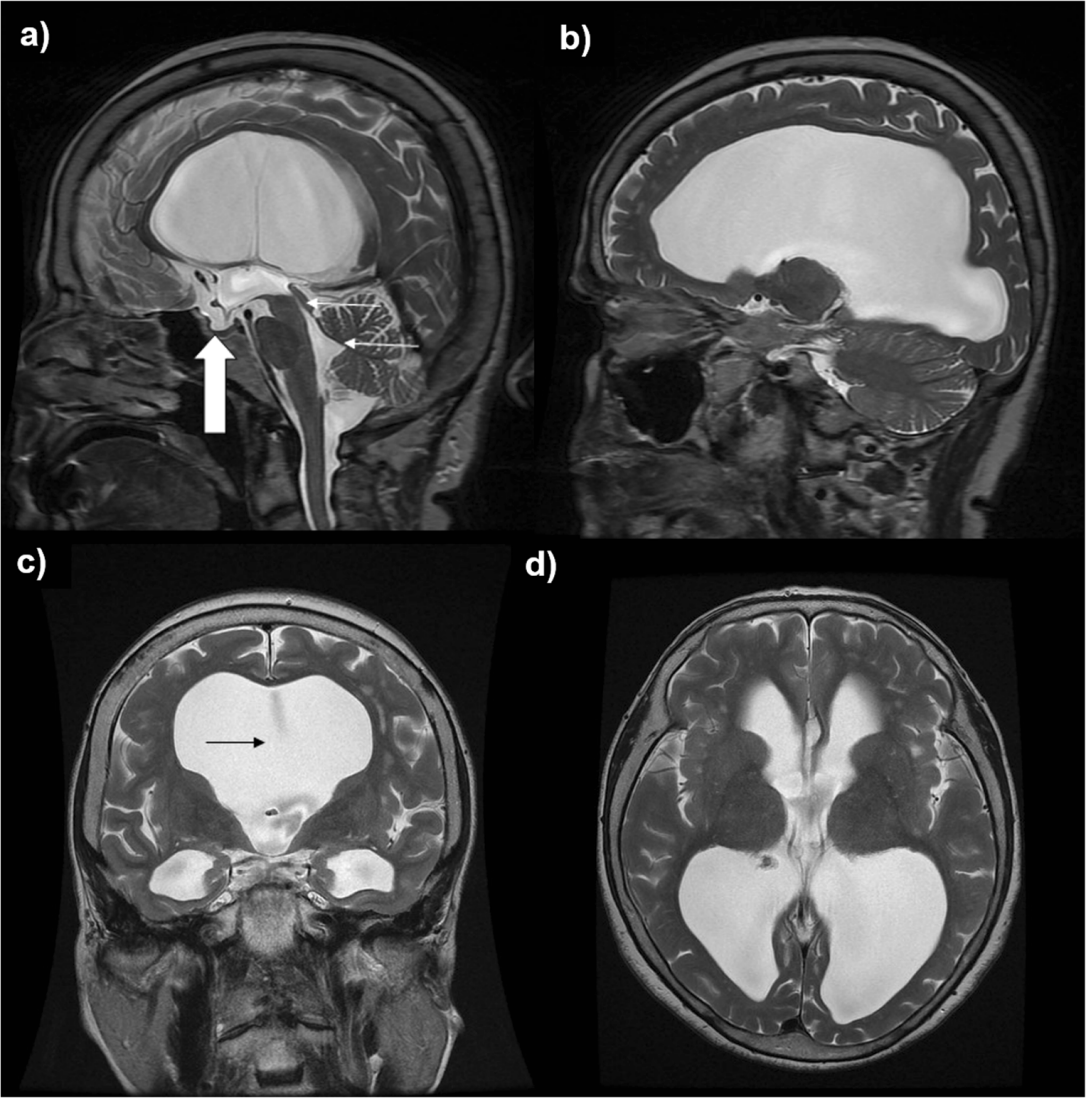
T2-weighted magnetic resonance images from a patient with LOVA: Case 14 in our case series. a and b Sagittal slices demonstrating triventriculomegaly, with sparing of the cerebral aqueduct and fourth ventricle (*thin white arrows*). There is apparent enlargement of the sella turcica due to the chronic nature of the hydrocephalus (*thick white arrow*). c Coronal slice demonstrating obliteration of the septum pellucidum (*black arrow*). The triventriculomegaly can once again be identified. d Subtle sulcal effacement may be appreciated, particularly in the right temporo-parietal region, on this axial slice from the same scan. However, this patient’s age-related (81) cerebral atrophy renders this effacement less marked than in typical cases of LOVA (where presentation is in the 5th–6th decades)
